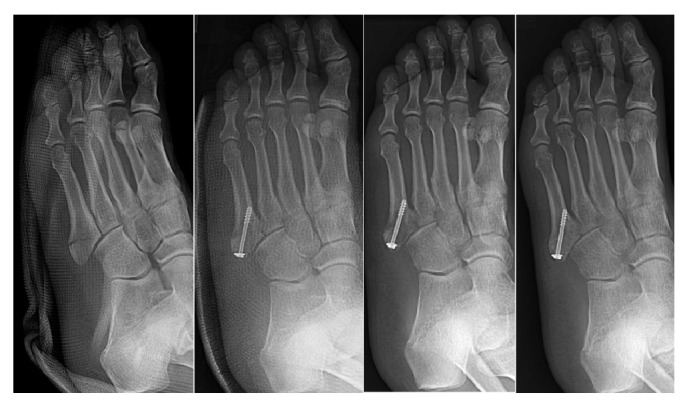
Operative treated, late weight-bearing patient's radiograph. 30-year-old male; radiograph of preoperation and 1-, 3-, and 6-month follow-up (from left to right).
